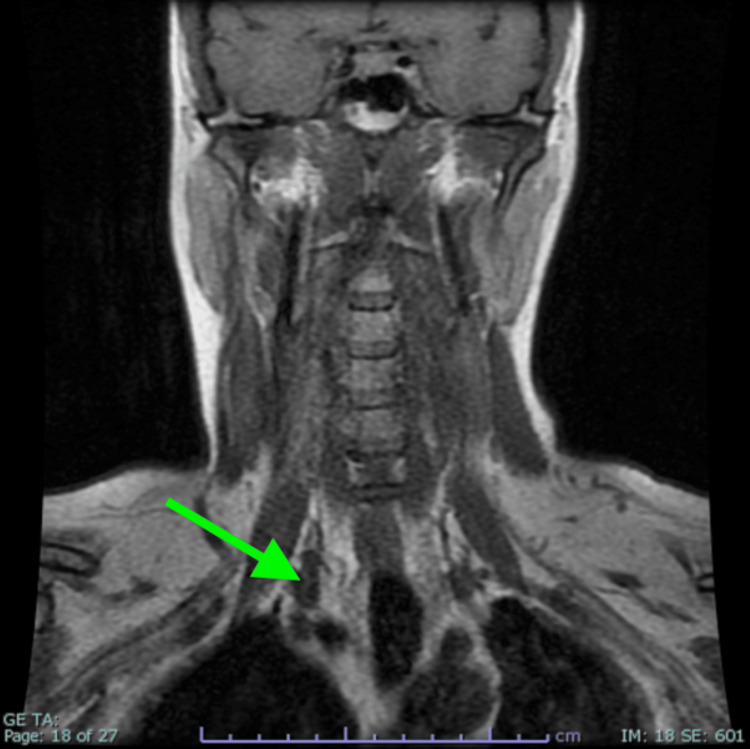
MRI post total thyroidectomy demonstrating resolution of tracheal midline shift, with an arrow identifying the surgical site of resection.
